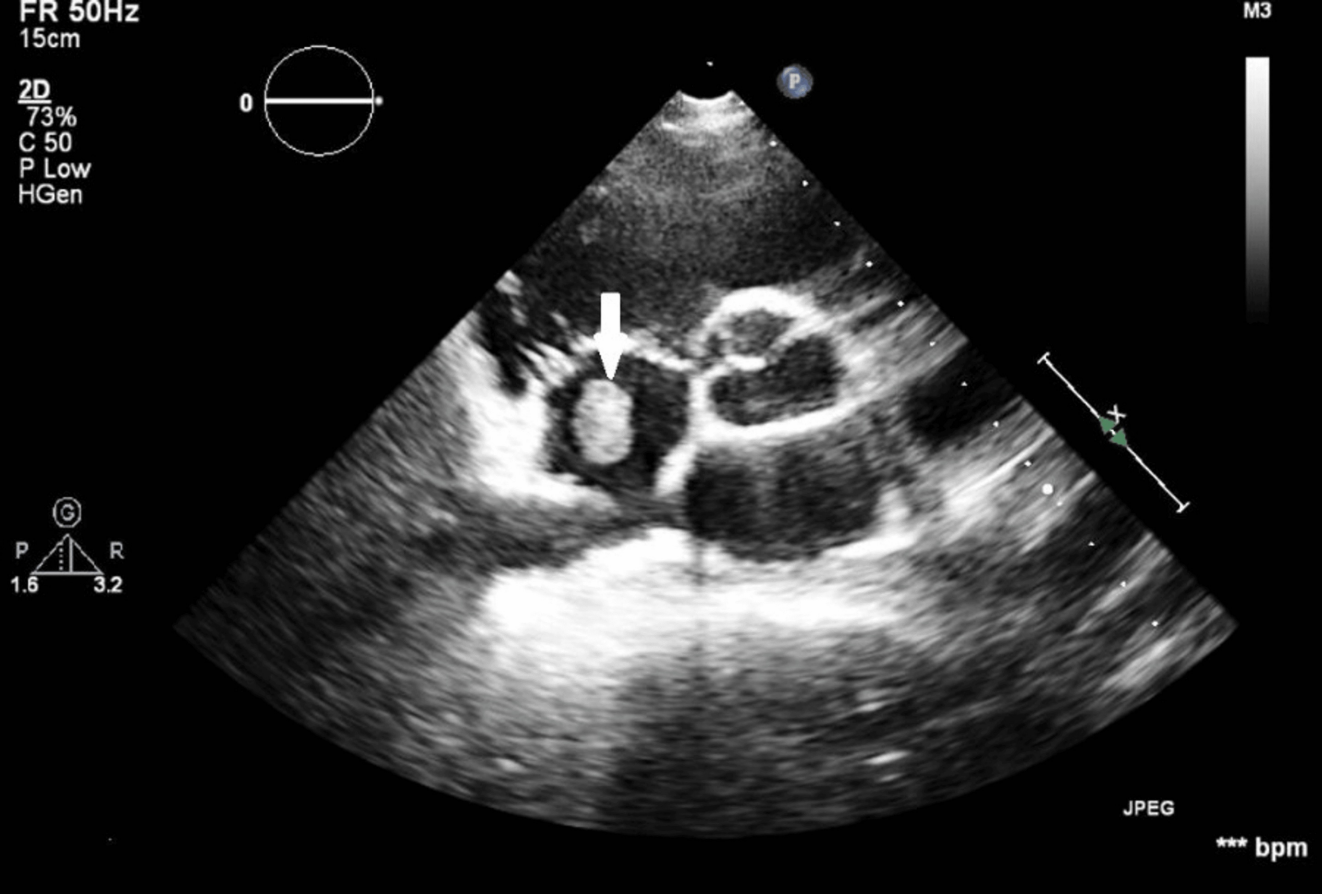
TTE in the short-axis view showing a round-shaped mass (white arrow) within the right atrium cavity. The mass measured 25x30 mm and is defined with a homogenous echotexture, suggestive of a possible thrombotic formation TTE, transthoracic echocardiogram
